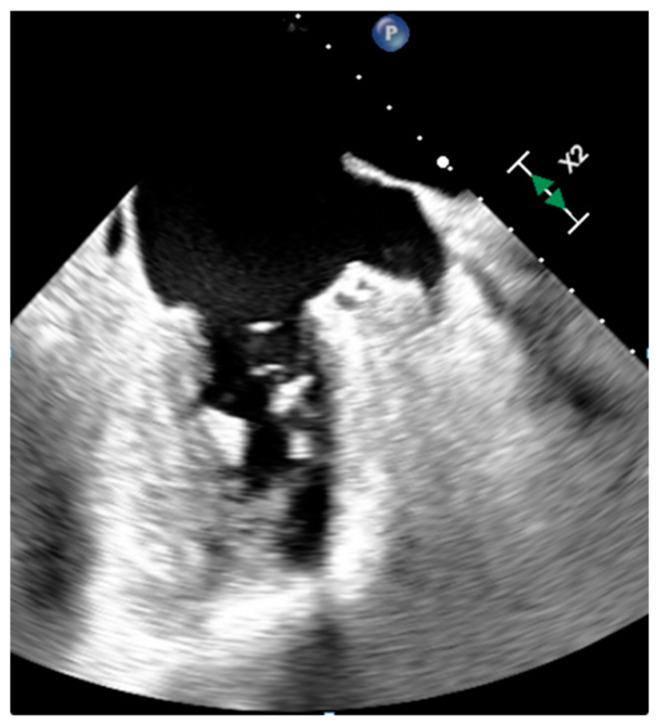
Warfarin ridge at transesophageal echocardiography.
